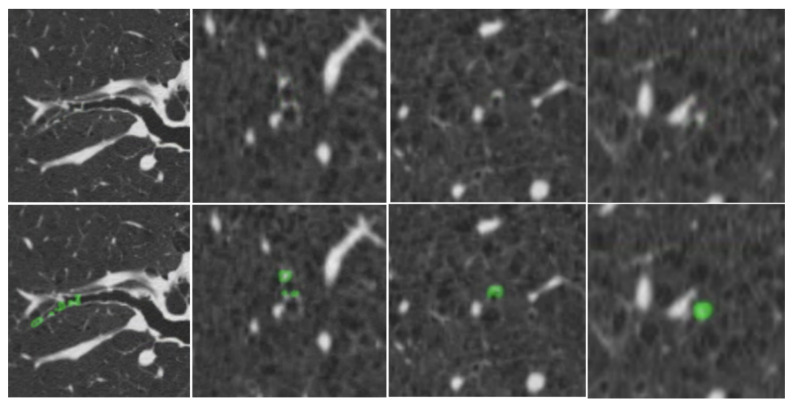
Examples of false negatives where both the S-Model and M-Model failed to detect the manually annotated regions. These misses typically occur in low-contrast regions or very small distal airways. Top row: original CT images. Bottom row: manually annotated mucus plugs (green).
